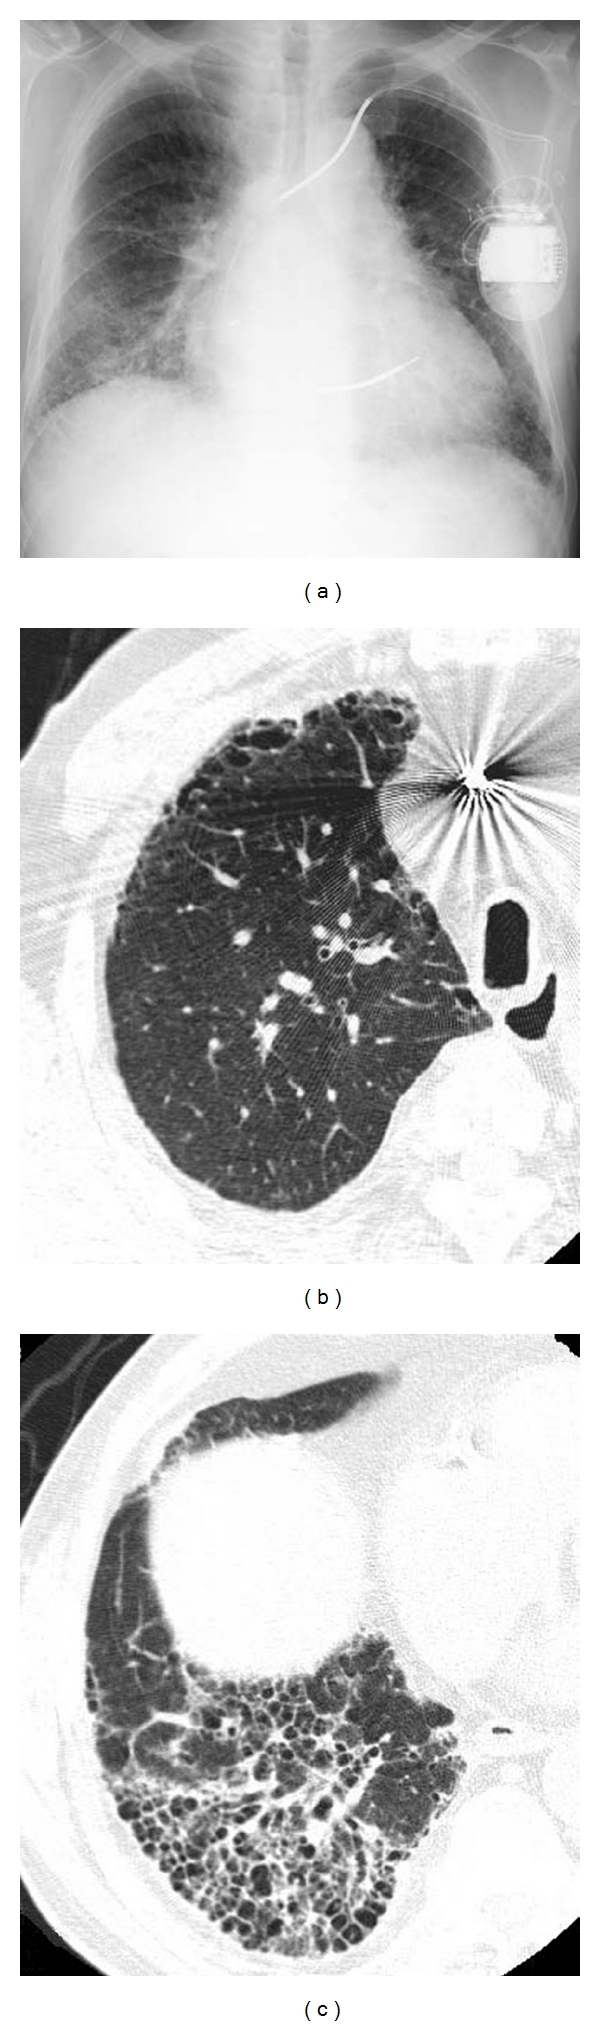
Diffuse interstitial pneumonia (UIP pattern) with emphysema. (a) Chest X-ray shows decreased volume of bilateral lungs. Ground glass opacity including reticular opacity and honeycomb lung in bilateral lower lungs. (b) HRCT of the right upper lobe shows paraseptal emphysema in ventral aspect. (c) HRCT of the right lower lobe shows ground glass opacity and honeycomb lung. Traction bronchiectasis is also observed within the areas of honeycombing.
